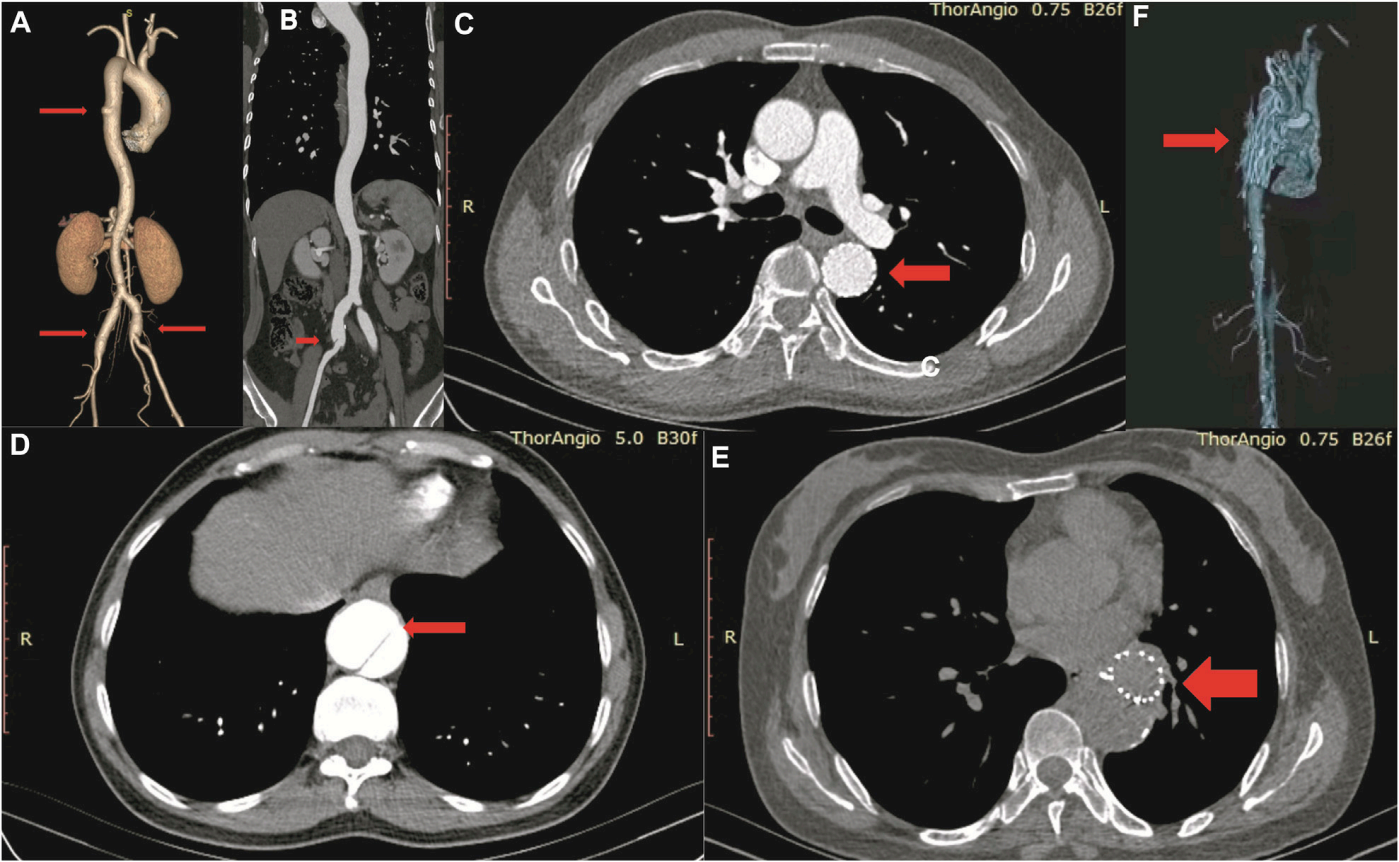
CT angiography image of III-4 in TAAD-1 (A, B), II-3 in TAAD-2 (C–E) and II-3 in TAAD-3 (F).
